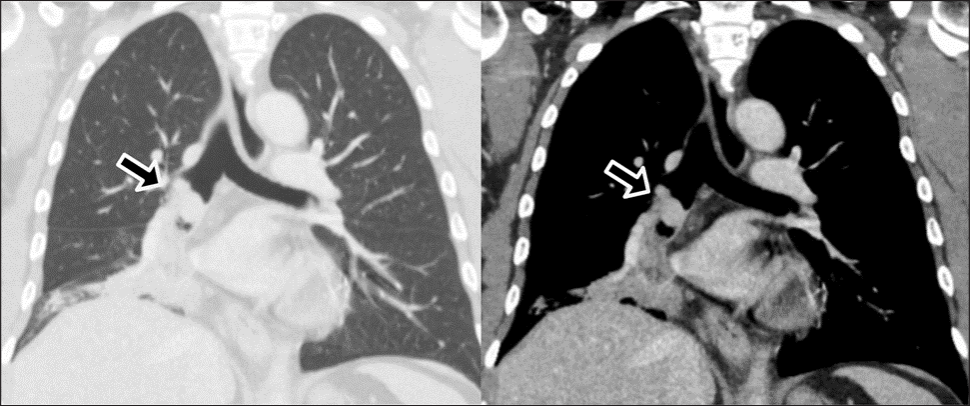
Coronal CT images demonstrating a spherical enhanced nodule (black arrow) in the bronchus intermedius causes obstructive pneumonitis in the medial segment of the right middle lobe and the basal segment of the right lower lobe.
